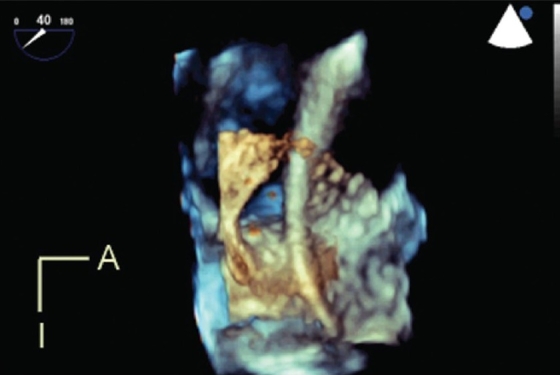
Live 3D transesophageal echocardiography demonstrates a catheter passing through a large atrial septal defect. The viewing perspective is unique: the observer is virtually located within the left atrium, looking rightwards. A, anterior; I, inferior
